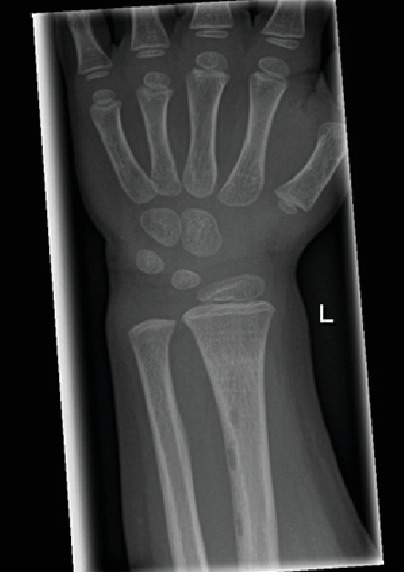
Anteroposterior radiograph showing complete consolidation and three well-circumscribed lucent lesions in the cortex, proximal to the previous fracture site.
